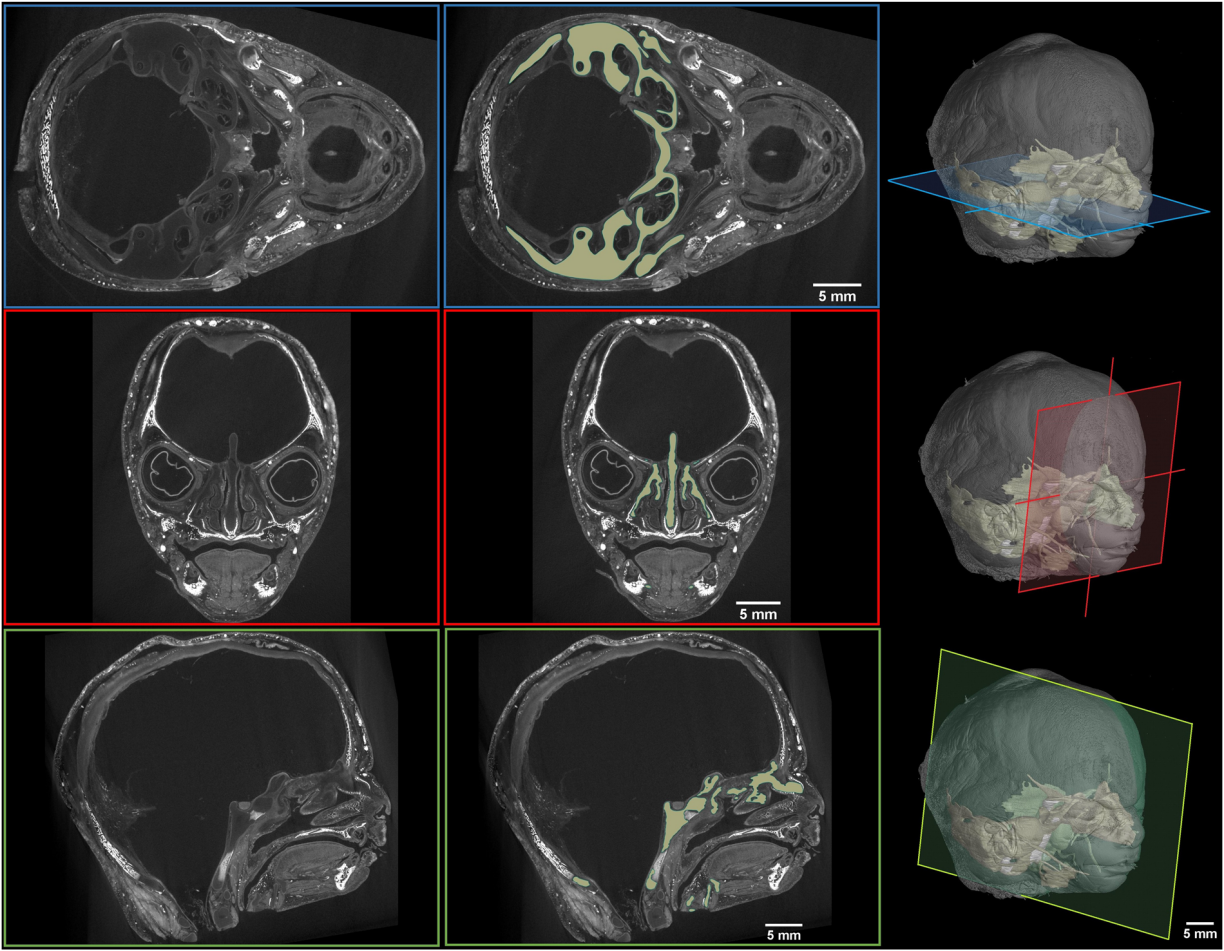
Validation of the segmentation on 14 weeks old sample. Color planes on the 3D models indicate the position of the CT slices: Transversal (blue), frontal (red) and sagittal (green) cross-section. Each cross-section show original raw CT slices (left) and the segmented chondrocranium (marked by brownish color in the right CT slice).
